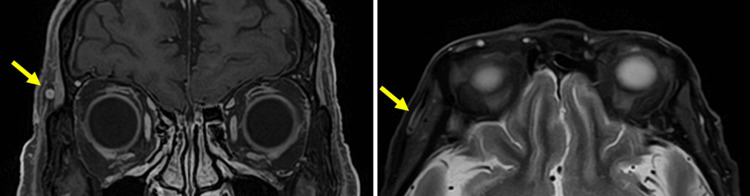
Orbital contrasted MRI images before treatment Left, T1 gadolinium-enhancing image: Right, STIR (Short Tau Inversion Recovery) image, Contrast enhancement was observed in the right superficial temporal artery.
